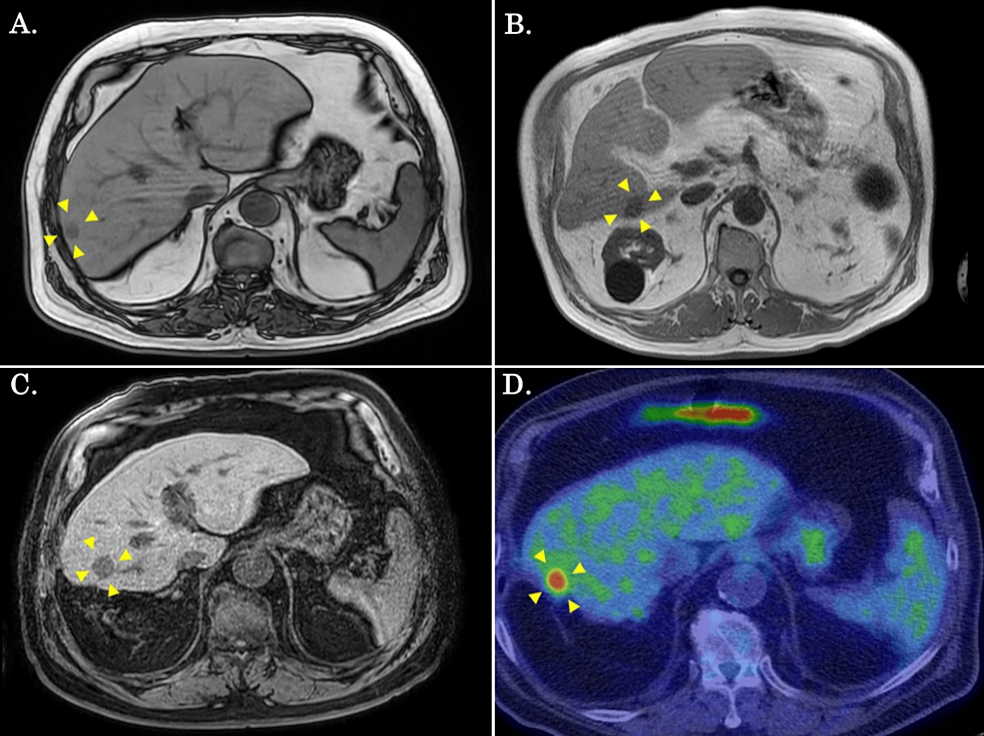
Preoperative findings at the following repeated liver resection in the current case A: MRI findings prior to laparoscopic S7/6 partial liver resection (the second operation). B: MRI findings prior to robotic S6 partial liver resection (the third operation). C: MRI findings prior to robotic S6 subsectionectomy (the fourth operation). D: FDG-PET findings prior to robotic S7 partial liver resection (the sixth operation). Arrowheads in each figure demonstrated the tumor. FDG-PET - ^18^F-fluorodeoxyglucose positron emission tomography
